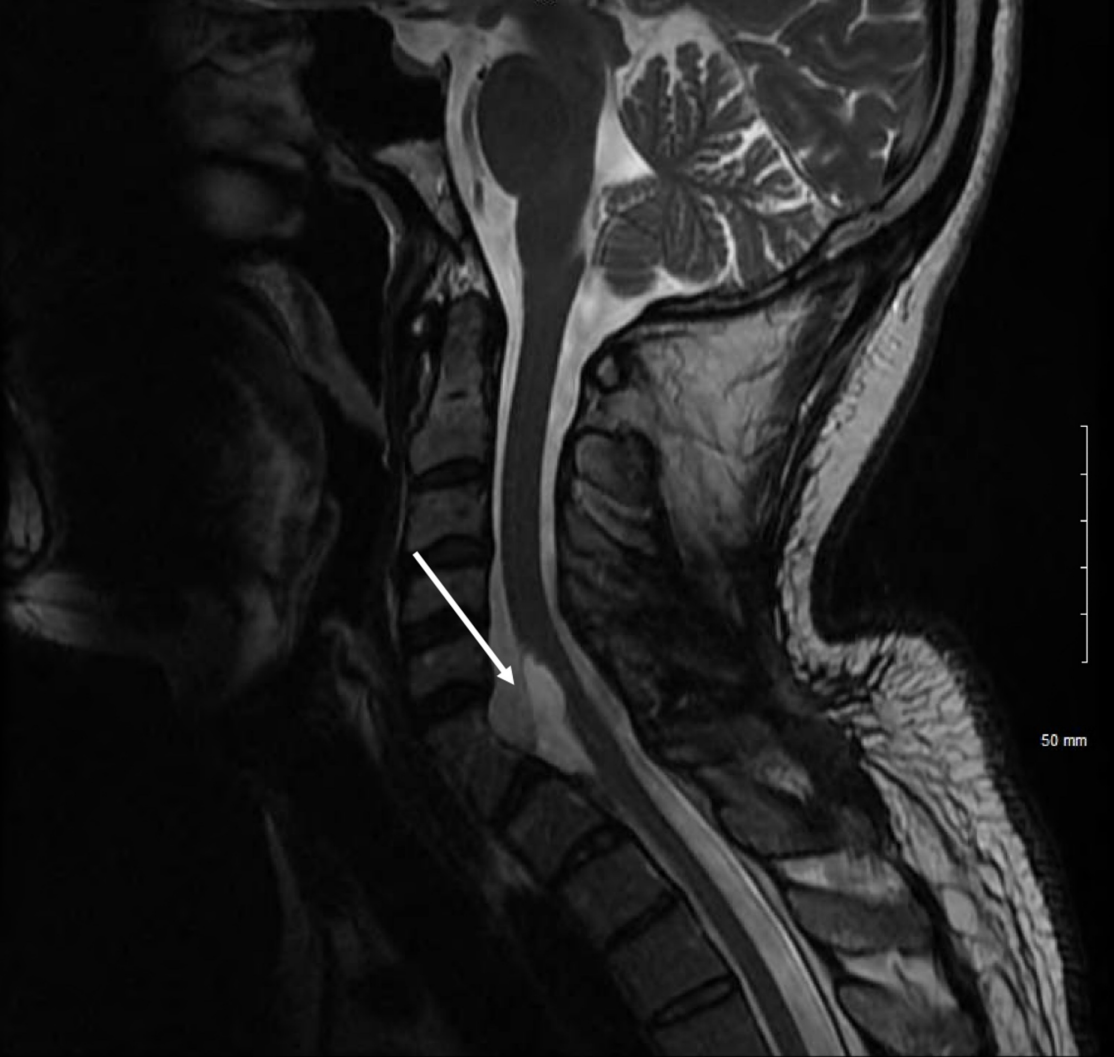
T2 MRI demonstrating residual cyst (white arrow) at six-month follow-up.
